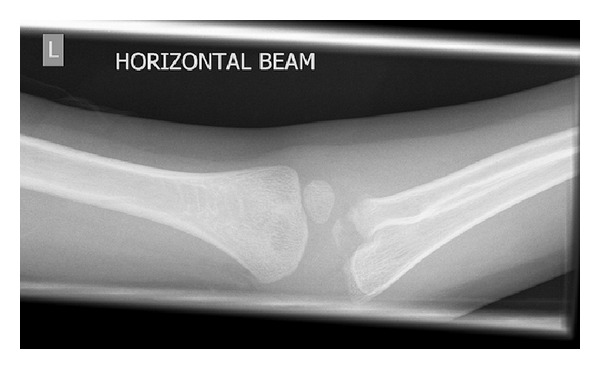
Anterioposterior radiograph: note the bony fragment and the medial radial head dislocation.
